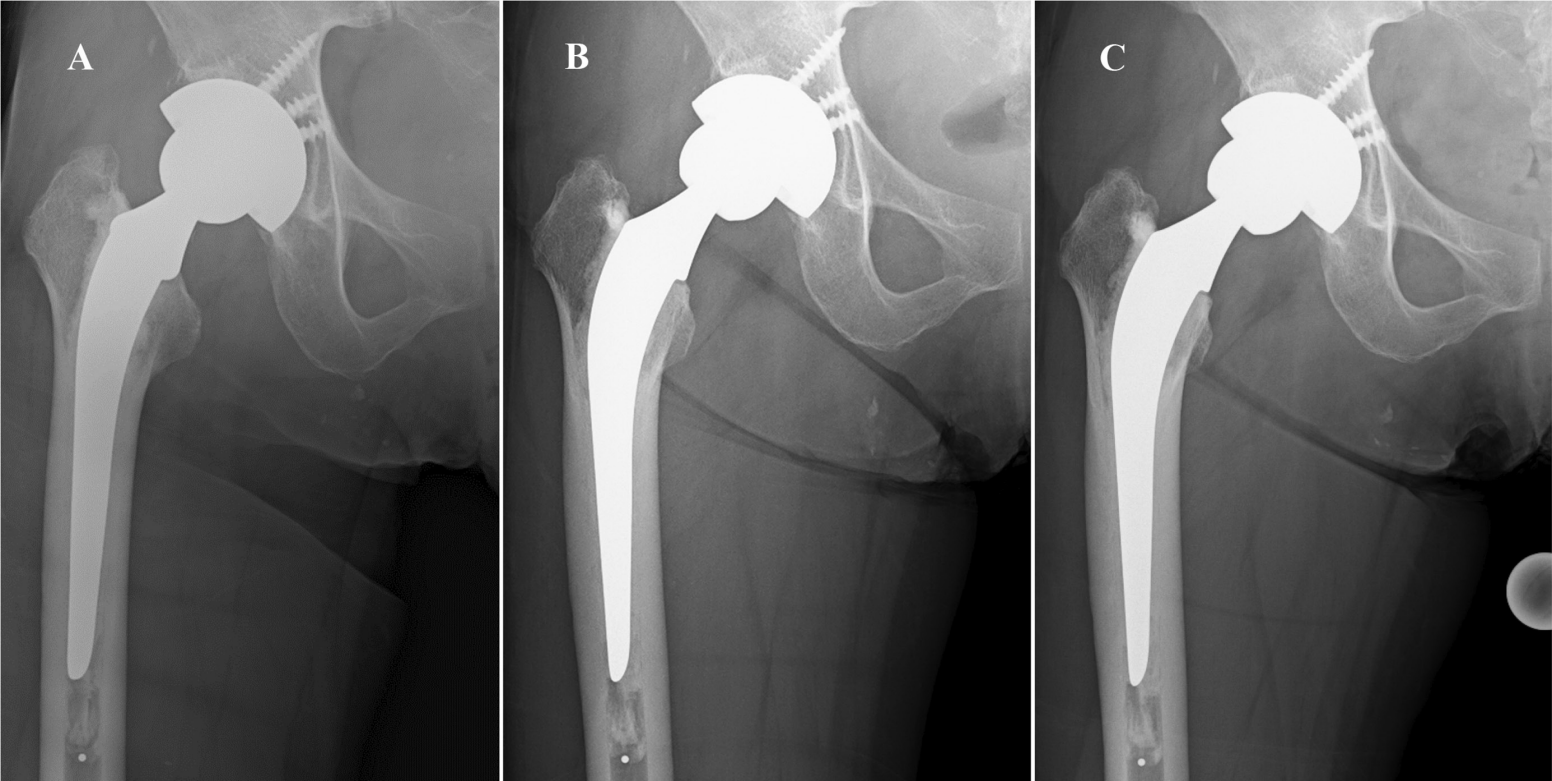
Radiographs after dislocation and closed reduction. Post‐reduction radiographs (A) showed that the femoral head was subtly eccentric compared with a radiograph after primary THA. Radiographs taken during a visit at a local hospital 3 months after dislocation revealed nonconcentric, incomplete reduction (B). In radiographs taken in our hospital, nonconcentric, incomplete reduction was more apparent (C).
